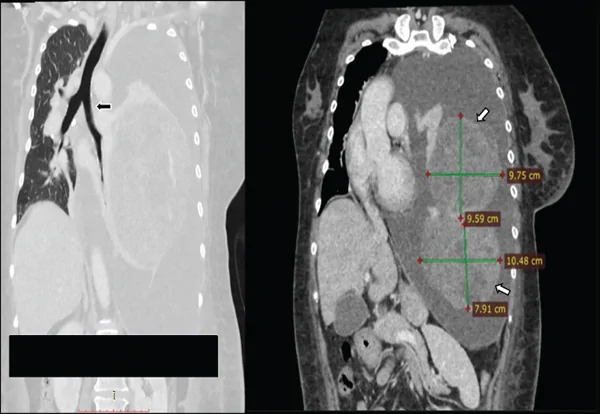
Coronal contrast‐enhanced chest CT demonstrating two giant pleural‐based masses with heterogeneous density and internal calcifications, causing extensive occupation of the left hemithorax and compression of adjacent thoracic structures. White arrows indicate the two masses, whereas the black arrow shows contralateral mediastinal and tracheal displacement. Maximum dimensions are indicated.
